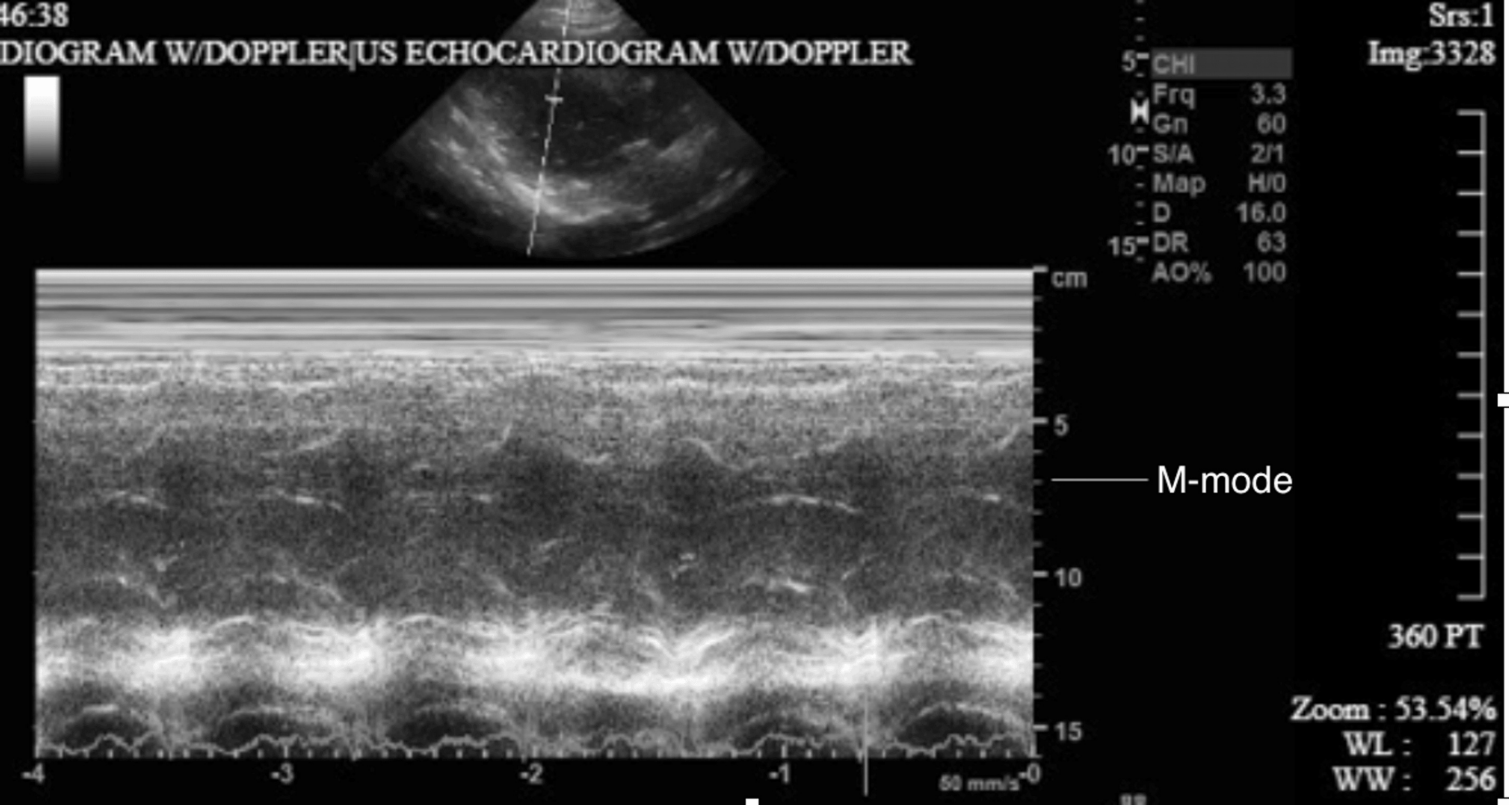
Transthoracic echocardiography showing preserved contractility on M-mode with left ventricular ejection fraction (LVEF) of 60-65%
